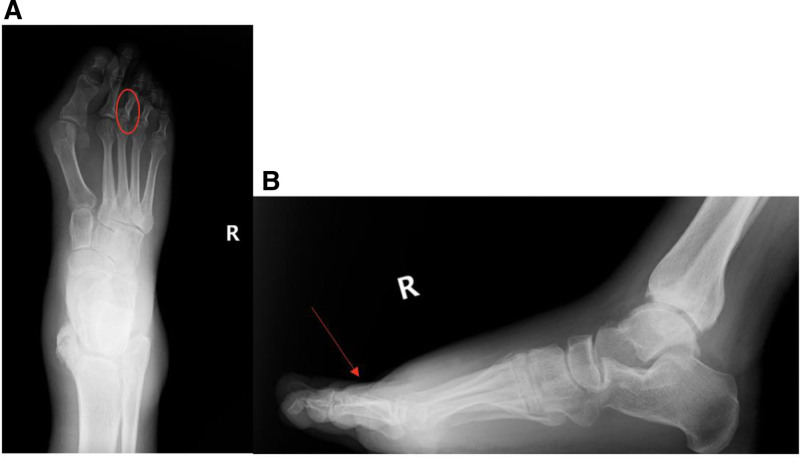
37-year-old male with a right foot abscess and a gradual onset of severe pain located on the medial instep of the right foot. Patient had X-ray foot right anterior-posterior and lateral imaging completed same day of admission. A 10 mm retained broken needle is positioned on the dorsal aspect of the proximal phalanx of the right third digit. The retained broken needle exhibits slightly enhanced visibility in the lateral film (B) compared the anterior-posterior film (A) in the setting of soft tissue inflammation.
